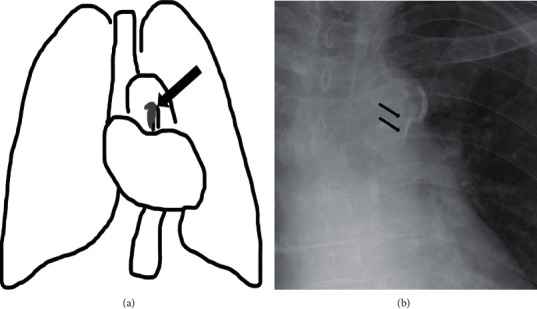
The scheme and representative X-ray images showing the Aortic Arch Tail Sign. The arrows show the calcification line of the ascending aorta adjacent to the aortic arch.
